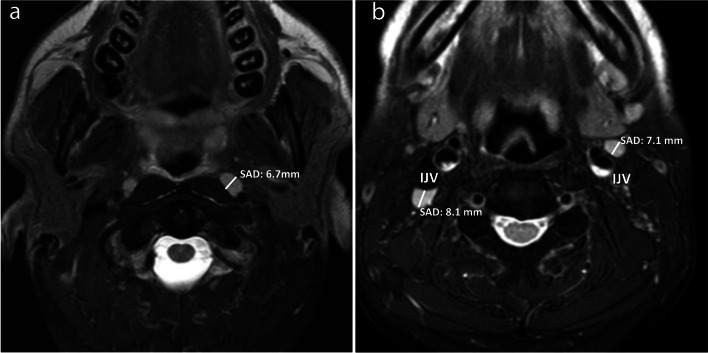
Axial T2-weighted fat-suppressed MR images of two patients without head and neck cancer showing bilateral normal/benign reactive retropharyngeal (a), left jugulodigastric (b) and right Level IIa (b) nodes. The mean SADs of the largest nodes for retropharyngeal, jugulodigastric, and Level IIa nodes were 3.9 ± 1.2 mm, 7.4 ± 1.9 mm, and 4.9 ± 1.6 mm, respectively. (IJV = internal jugular vein, SAD = short axis diameter)
